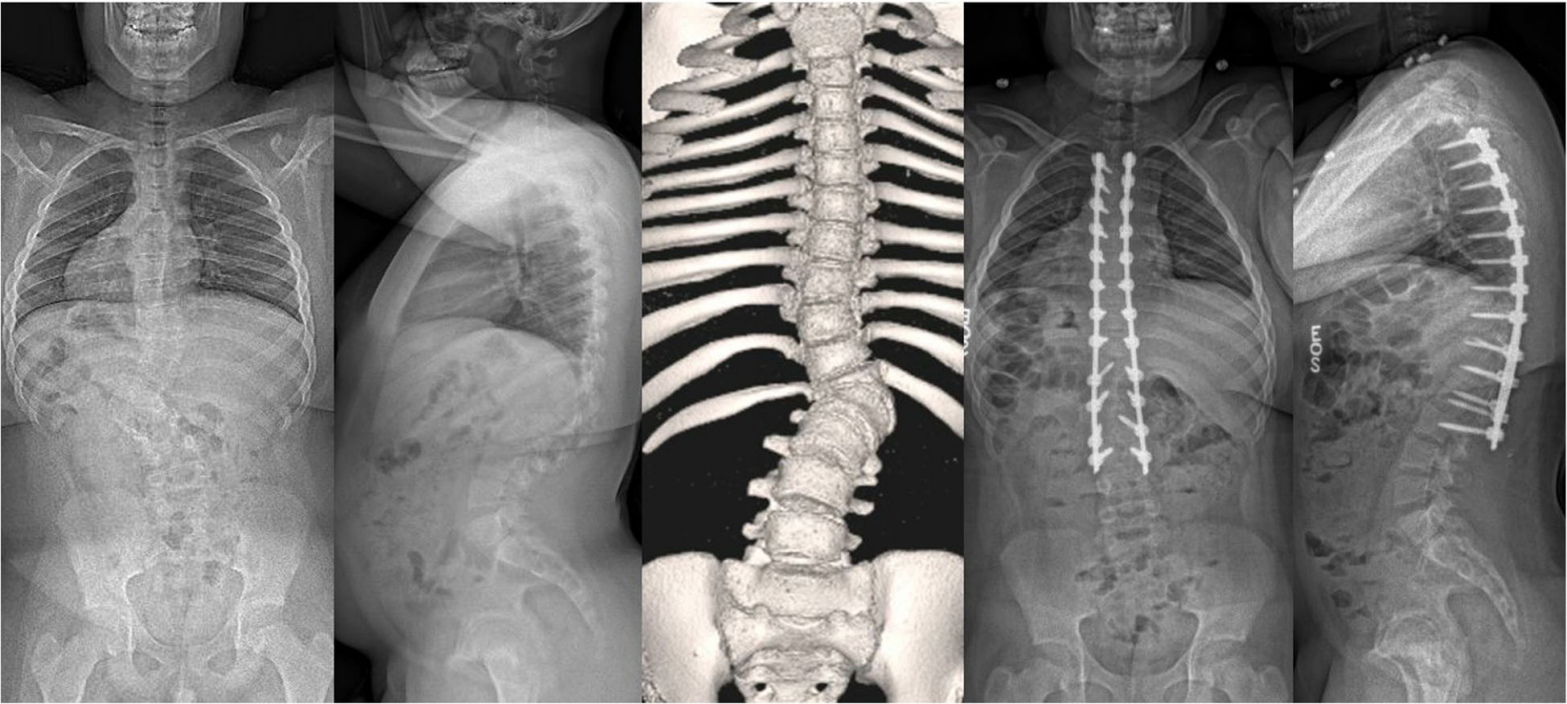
Pre- and 2-year postoperative anteroposterior and lateral radiographs and 3D CT of a 13-year-old male with congenital scoliosis treated by posterior spinal fusion with L1-hemivertebra resection. Intraoperative blood loss was 100 mL. The 2-year postoperative radiographs show presence of radiographic distal junctional kyphosis which remains asymptomatic
